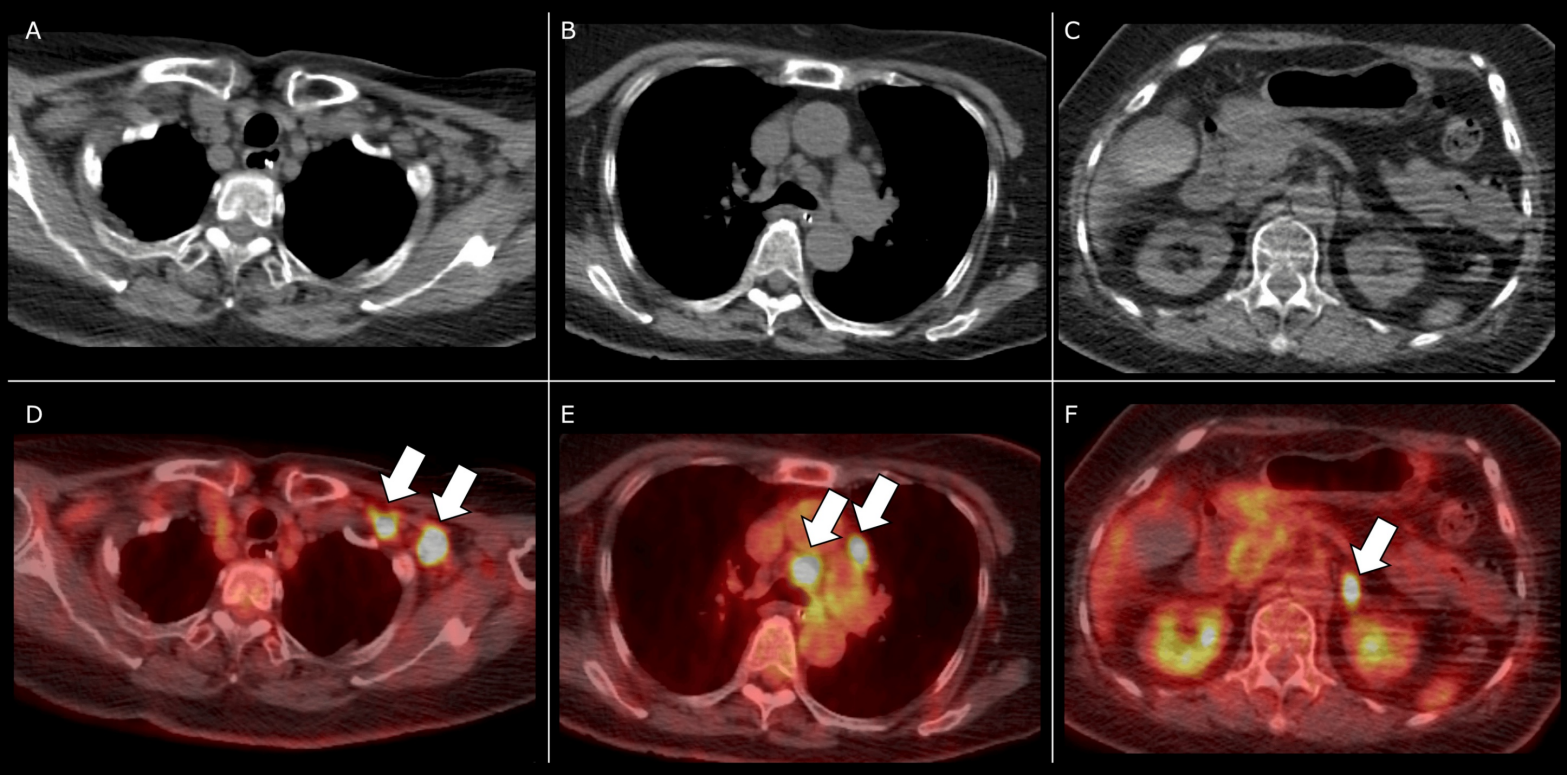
CT and positron emission tomography/CT There was intensive 18F-fluorodeoxyglucose uptake at mediastinal lymph nodes, supraclavicular lymph nodes, and the left adrenal gland (arrow). CT: computed tomography
